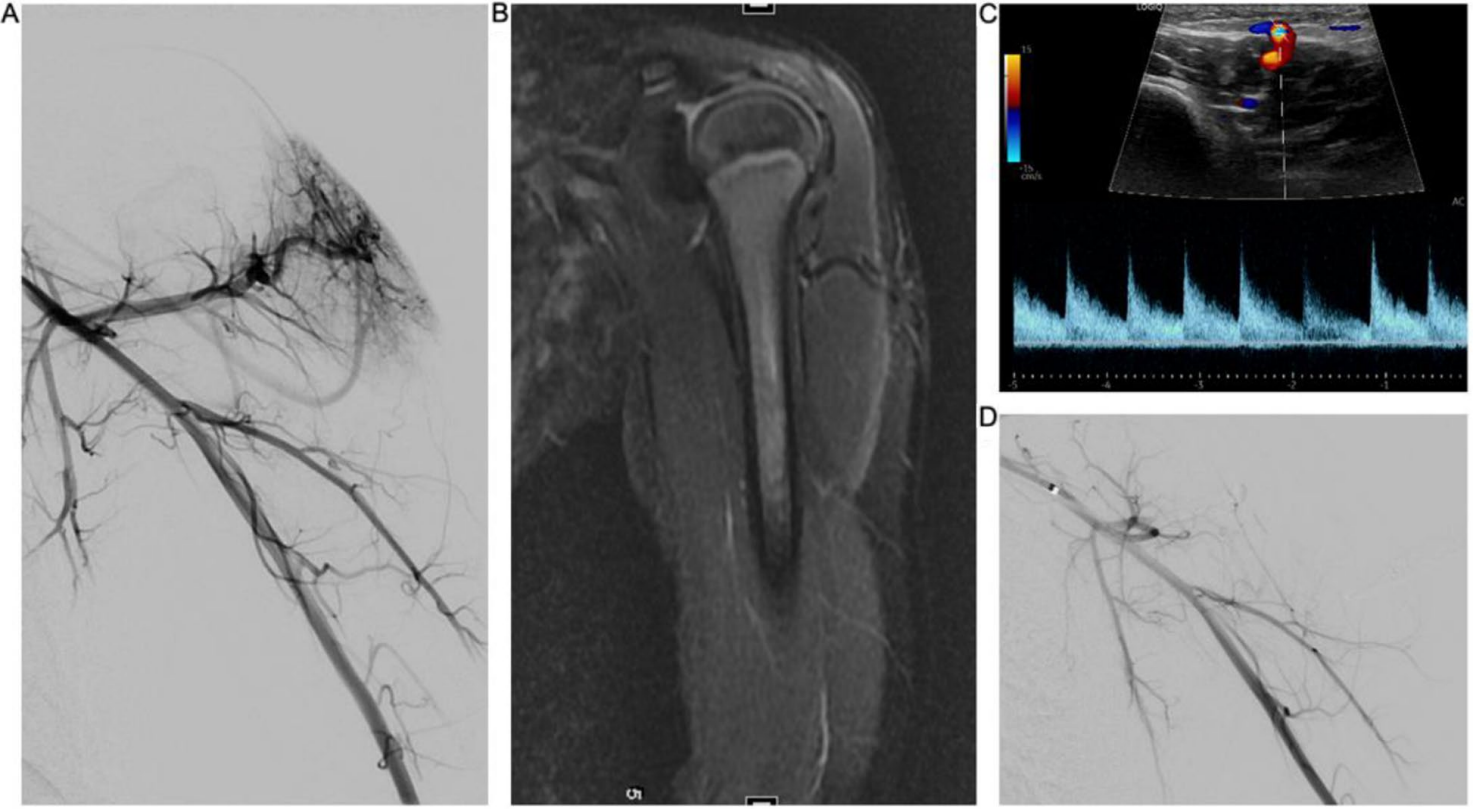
Shoulder AVM with overlying skin changes. A An angiogram from the axillary artery showing an arteriovenous malformation centered over the deltoid. B T2 coronal fat sat MRI showing a vessel traversing the deltoid with smaller branches in the subcutaneous tissue. C Ultrasound shows a large arterial pedicle traversing the deltoid and feeding branches in the subcutaneous tissue. D. Angiogram post-embolization with N-BCA from the axillary artery
